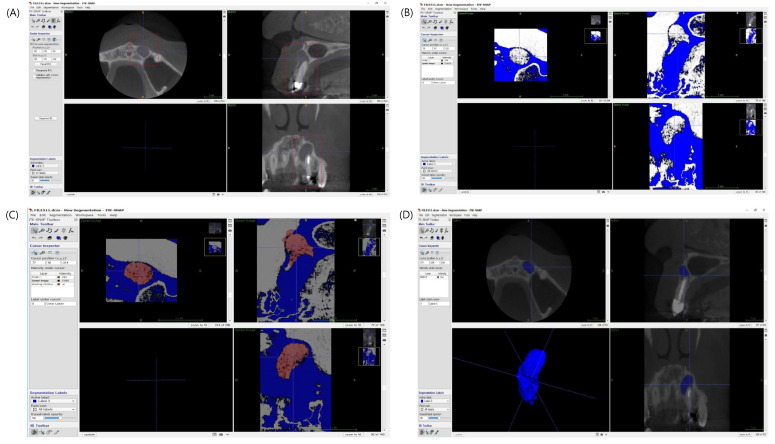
Lesion volume calculation using ITK-SNAP. (A) manual segmentation of a periapical defect in sagittal, axial and coronal views. (B) Selection of periapical defects using a grayscale value range selection tool. (C) Semiautomatic defect volume recognition and (D) reconstruction of 3D image on a lesion and calculation of the lesion volume.
